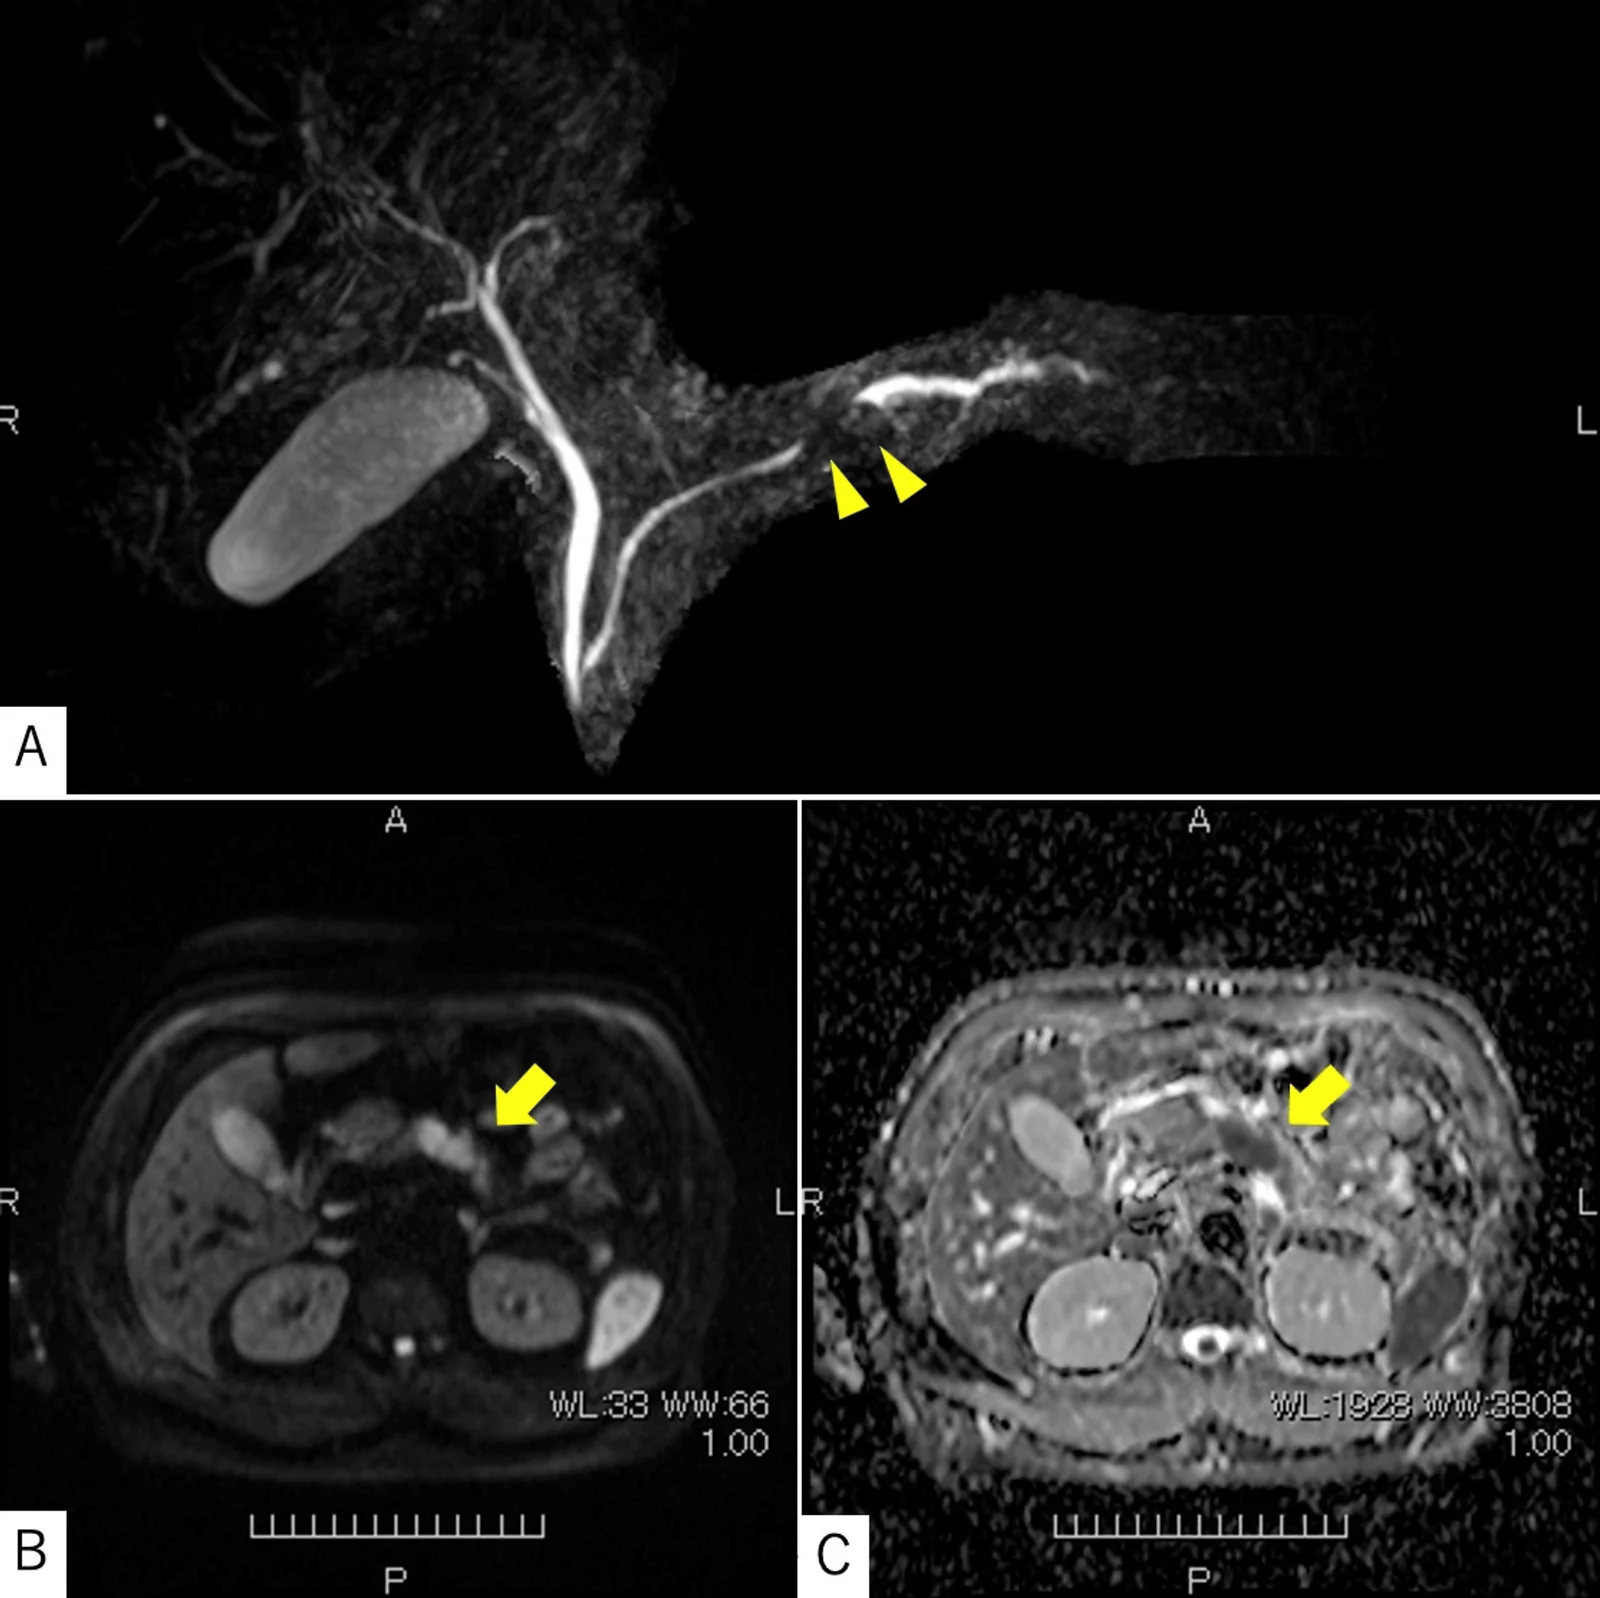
Abdominal magnetic resonance imaging findings at initial presentation (Day 1) (A) Magnetic resonance cholangiopancreatography demonstrating mild dilation of the distal main pancreatic duct (arrowheads). (B) Diffusion-weighted imaging showing a 4 × 2 cm mass in the pancreatic body with high signal intensity (arrow). (C) An apparent diffusion coefficient map revealing a corresponding signal reduction in the same lesion (arrow)
